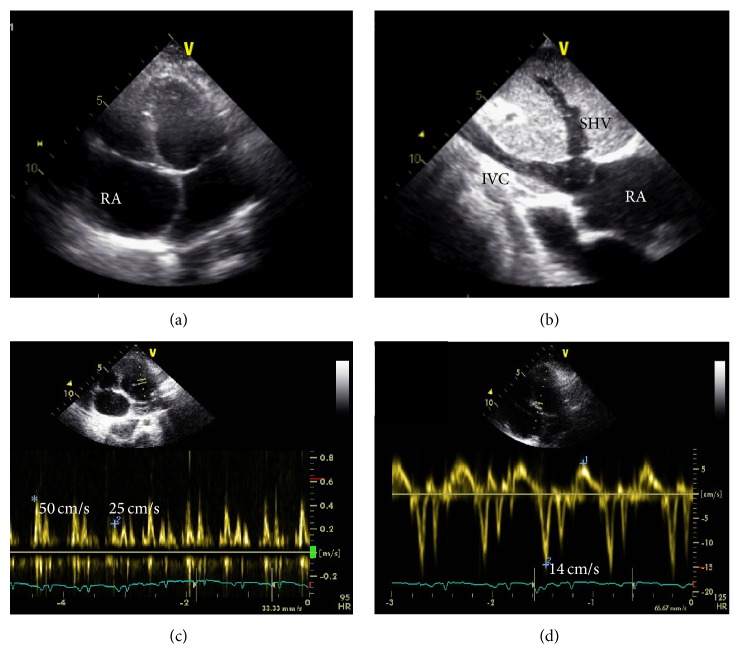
(a) Apical 4-chamber view with dilated right atria (RA), left deviated atrial septum; (b) subcostal view of dilated inferior vena cava (IVC) and suprahepatic veins (SHV); (c) significant (50%) transmitral flow variation with breathing, increasing during expiration (*∗*) and decreasing during inspiration (+); (d) high protodiastolic mitral annulus velocity (septal 14 cm/s, higher than lateral).
